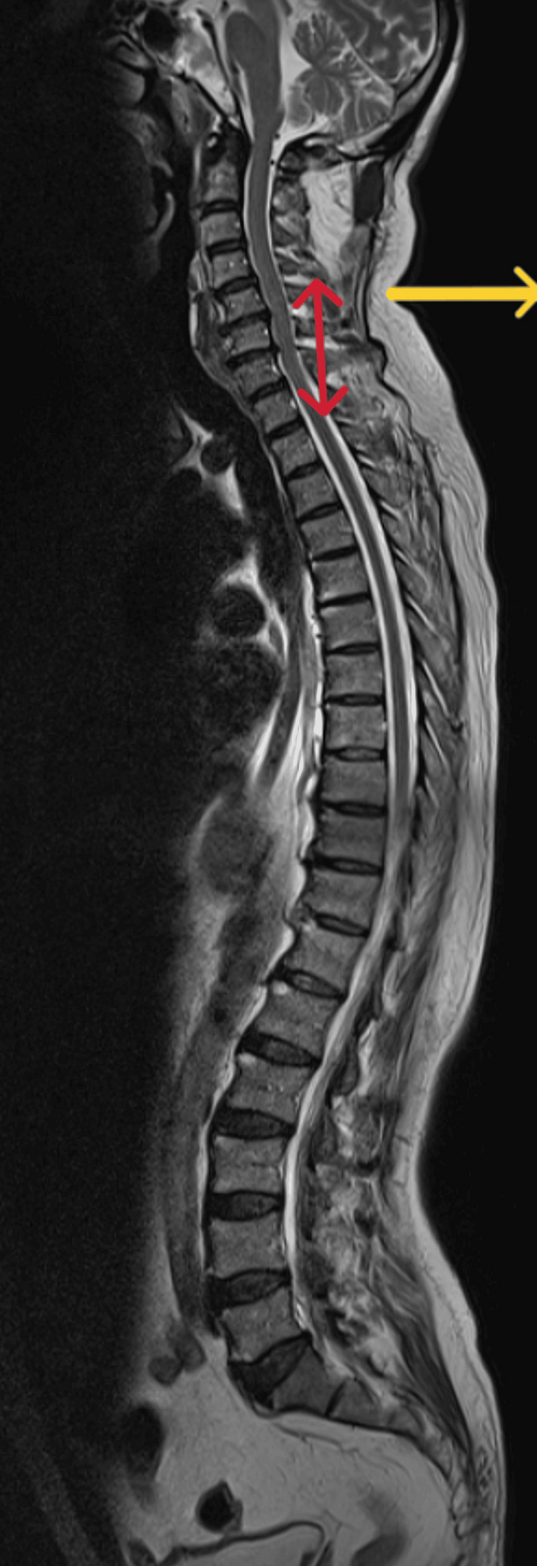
Magnetic resonance imaging of whole spine (axial view) Red arrow indicates posterior disc bulge at C4-C5, C5-C6, C6-C7. Yellow arrow indicates loss of cervical lordosis.
